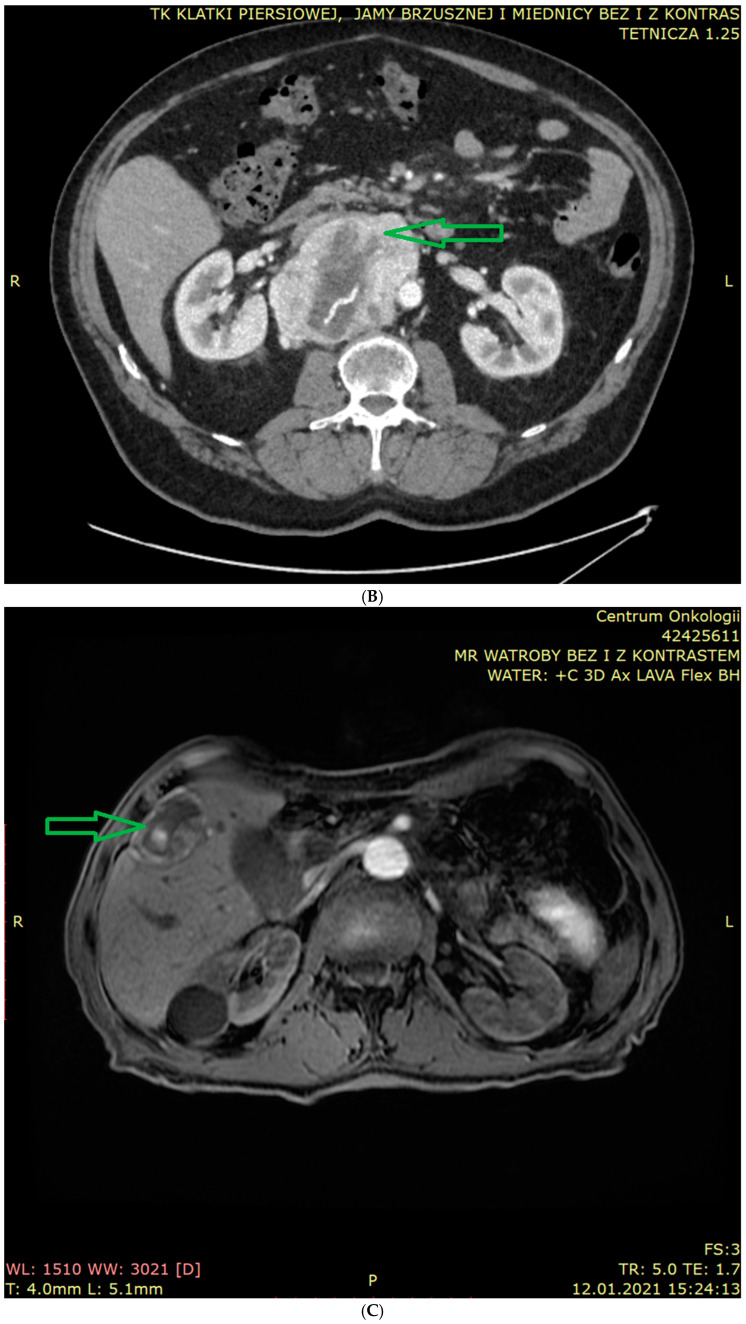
(A) PET-CT scan of bilateral neck masses (carotid body tumors, CBT) in a 31-year-old white female patient with familial PPGL1 syndrome—SDHD C11X. (B) CT scan of retroperitoneal paraganglioma in a 50-year-old white male patient. (C) MRI scan of metastatic pheochromocytoma (liver metastasis) in a 73-year-old white male patient.
